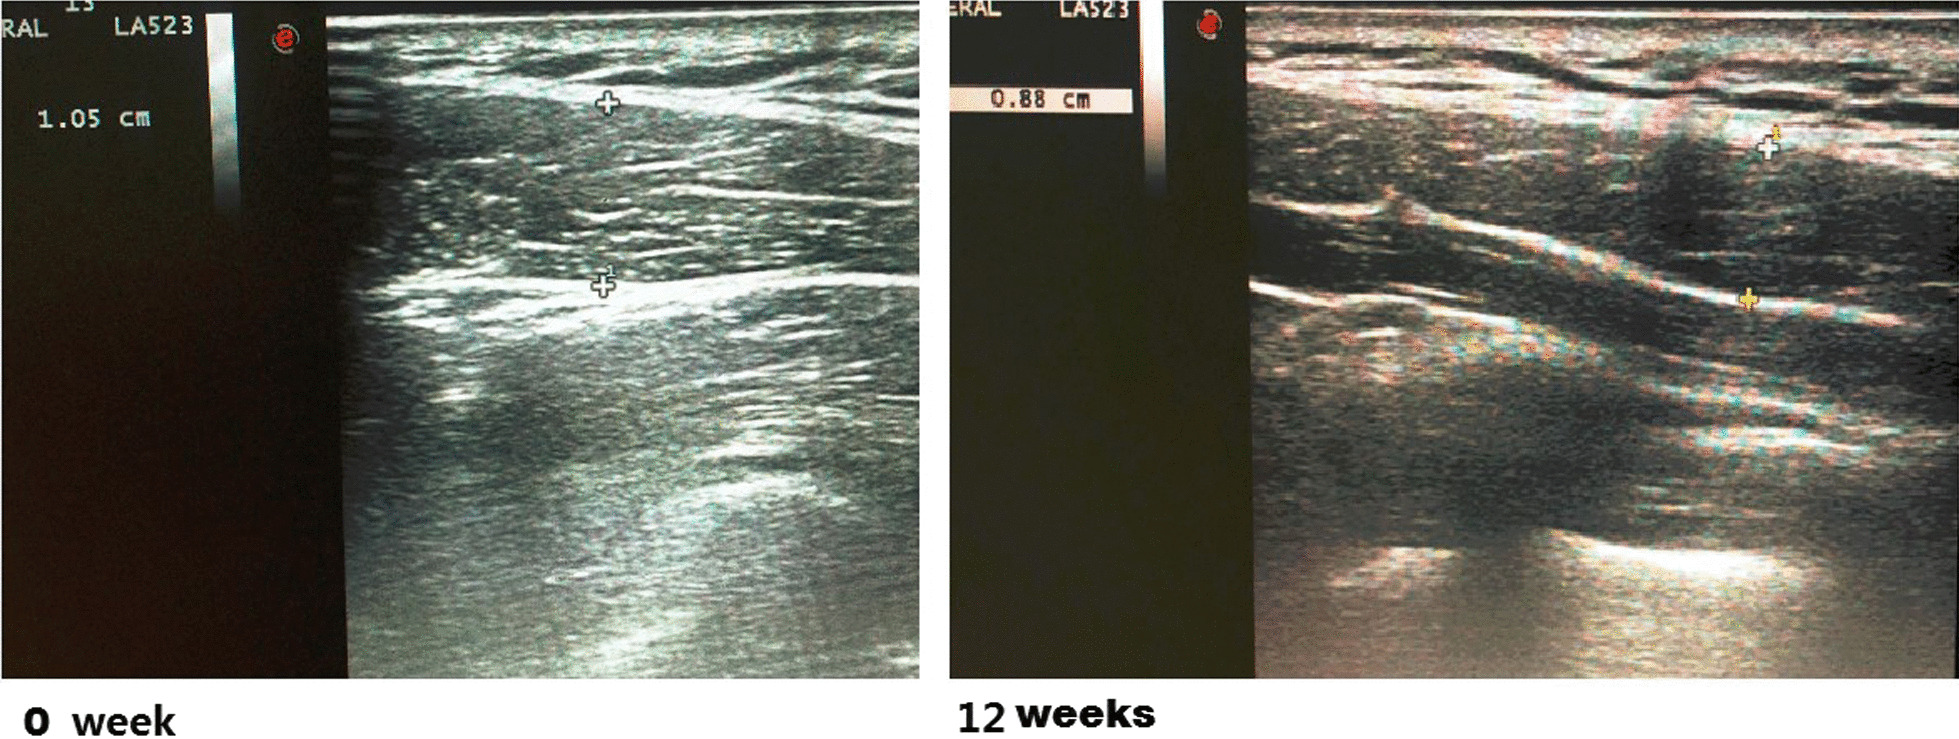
The muscle thicknesses before and after surgery were measured by using the ultrasound scanner
